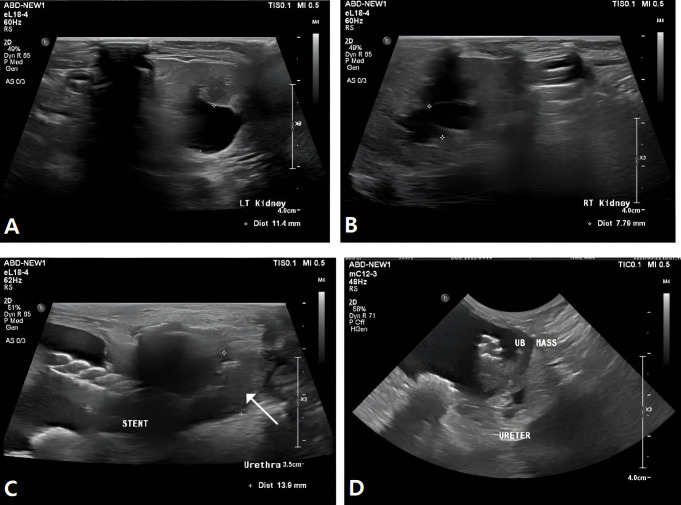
Follow-up ultrasonographic findings demonstrating disease progression and bilateral hydronephrosis. (A) Ultrasonographic image of the left kidney showing renal pelvic dilation (11.4 mm), consistent with hydronephrosis. (B) Ultrasonographic image of the right kidney demonstrating renal pelvic dilation (7.7 mm). (C) Ultrasonographic image showing tumor invasion (arrow with line) into the urethra distal to the caudal margin of the urethral stent. (D) Ultrasonographic image demonstrating marked progression of the mass, with increased thickness up to 24 mm.
